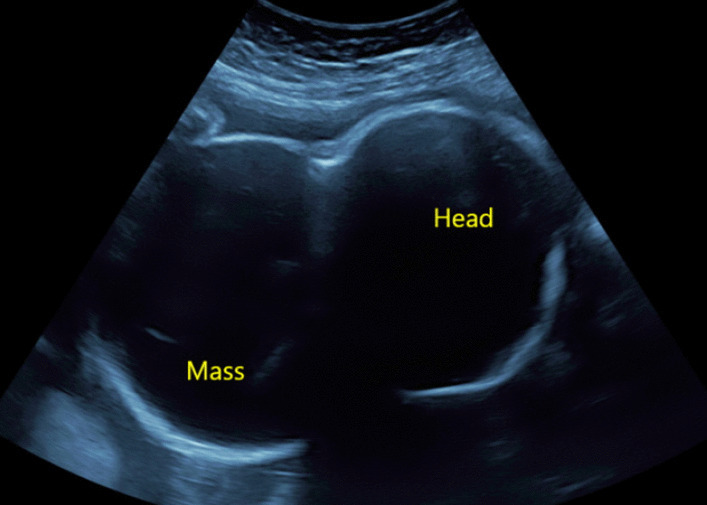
Ultrasound showing the enlarged lesion at 30 + 2 weeks gestation. This shows an ultrasound image of the mass at 30 + 2 weeks gestation. The tumour now measures 9 × 9 × 10 cm
